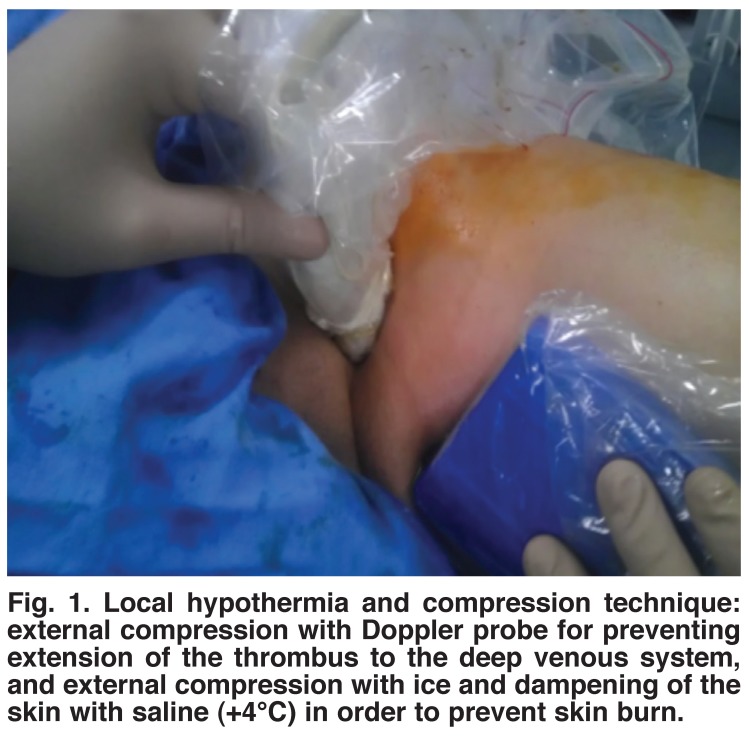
Local hypothermia and compression technique: external compression with Doppler probe for preventing extension of the thrombus to the deep venous system, and external compression with ice and dampening of the skin with saline (+4°C) in order to prevent skin burn.
